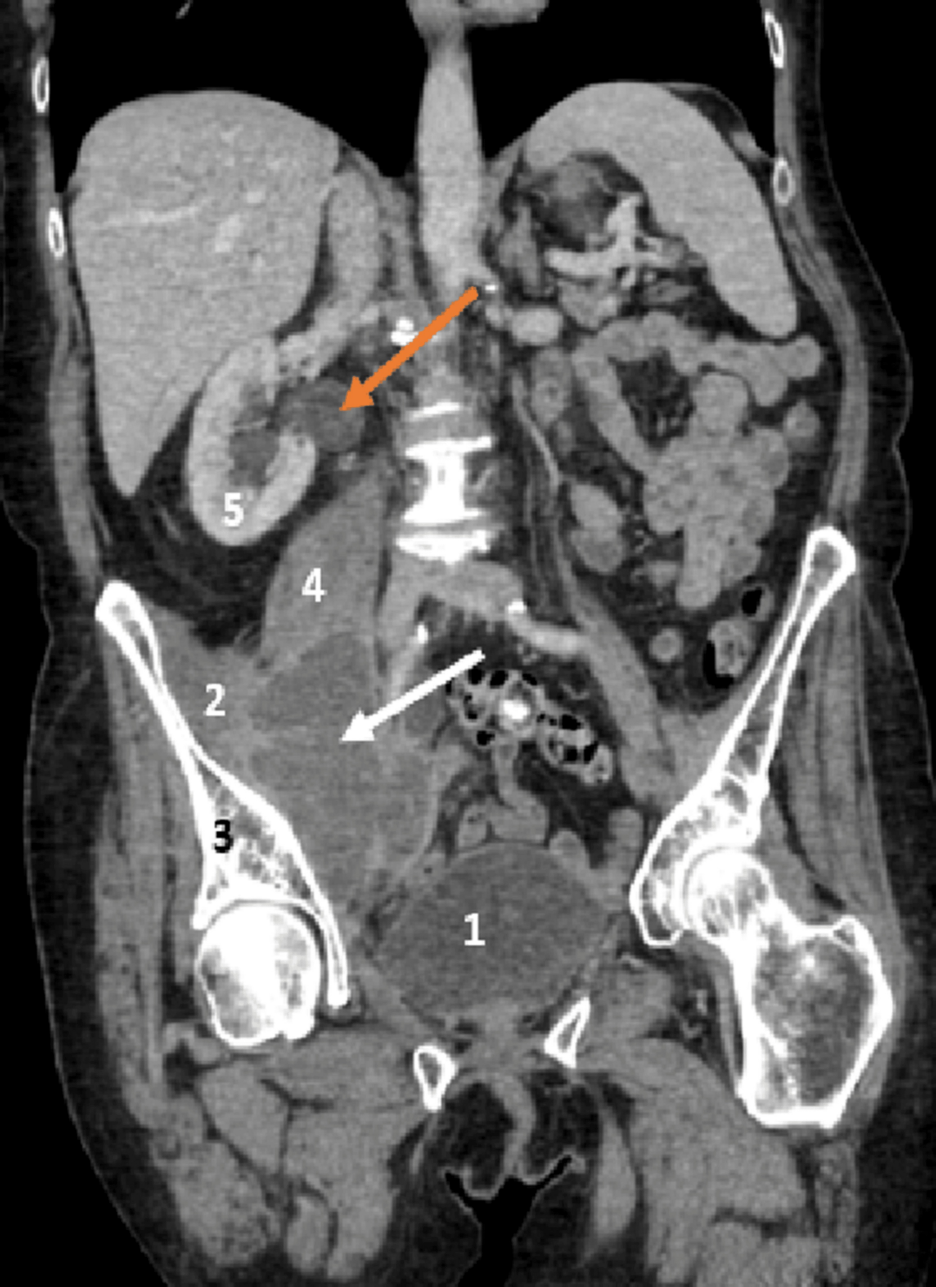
Contrast-enhanced computed tomography abdomen and pelvis coronal view 1. Bladder, 2. Iliacus muscle, 3. Pelvis, 4. Psoas muscle, 5. Right kidney White arrow: Psoas abscess; Orange arrow: Hydroureteronephrosis
